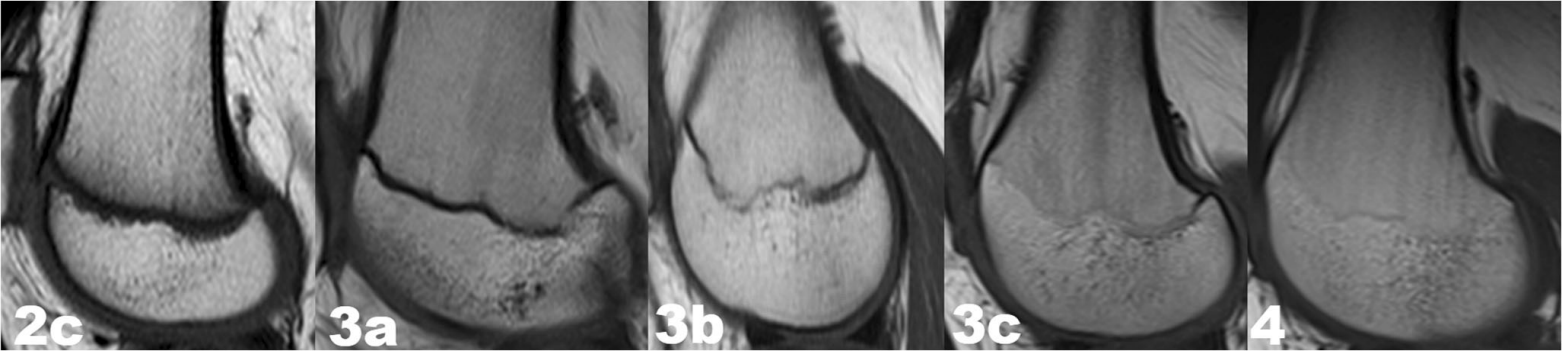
T1-weighted turbo spin echo (T1-TSE) sequences in the sagittal plane on MRI images: stages 2c,3a,3b,3c, and 4 according to combined scoring system described by Schmeling et al. [21] and Kellinghaus et al. [22] for distal femoral epiphysis
